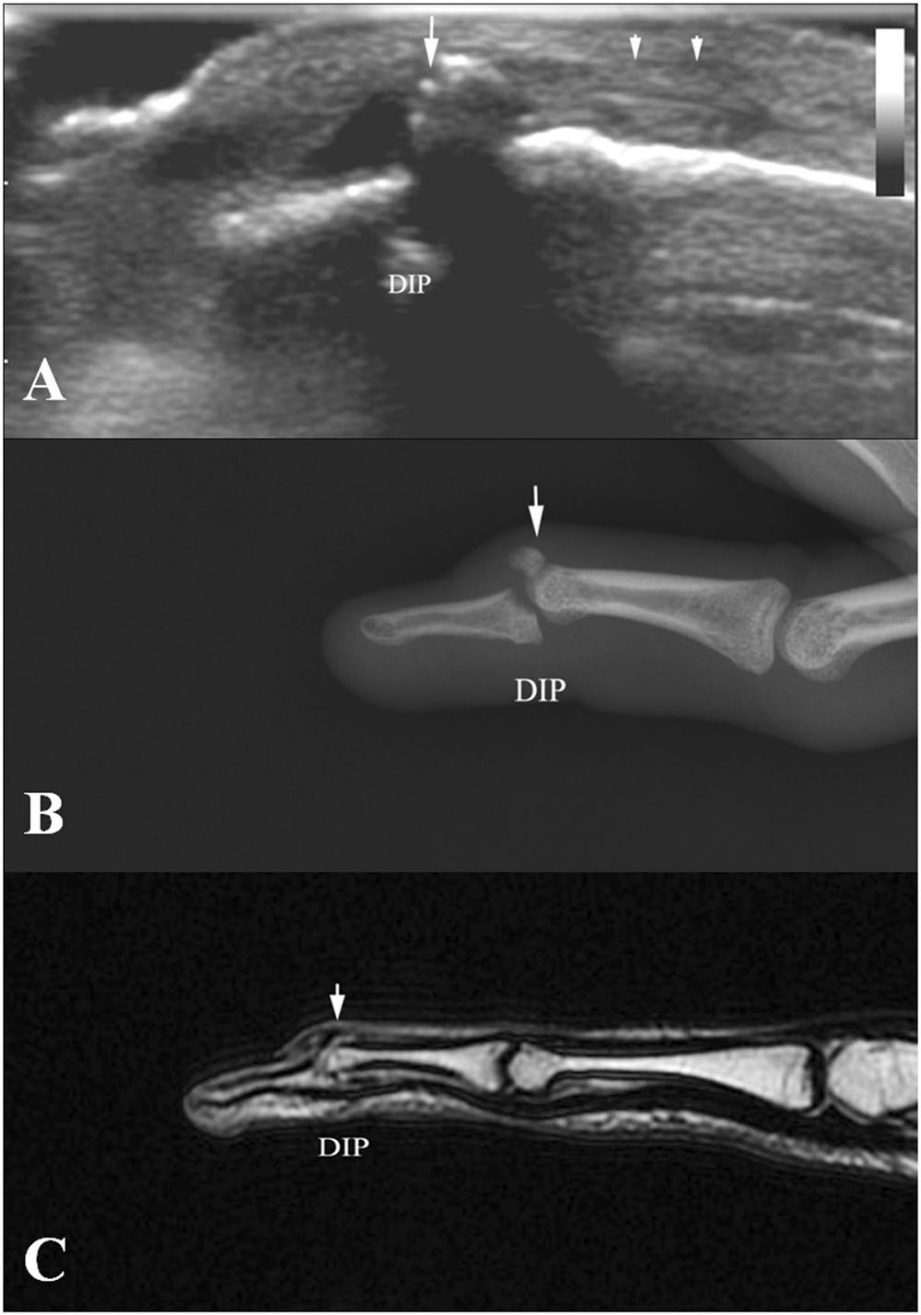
Type A mallet finger. High frequency ultrasonography, X-ray and MR images of type A mallet finger inury. Figure 2A: The retracted extensor tendon (short arrow), the avulsion bony fragment (long arrow); Fig. 2B and Fig. 2C: The avulsion bony fragment (arrow).
